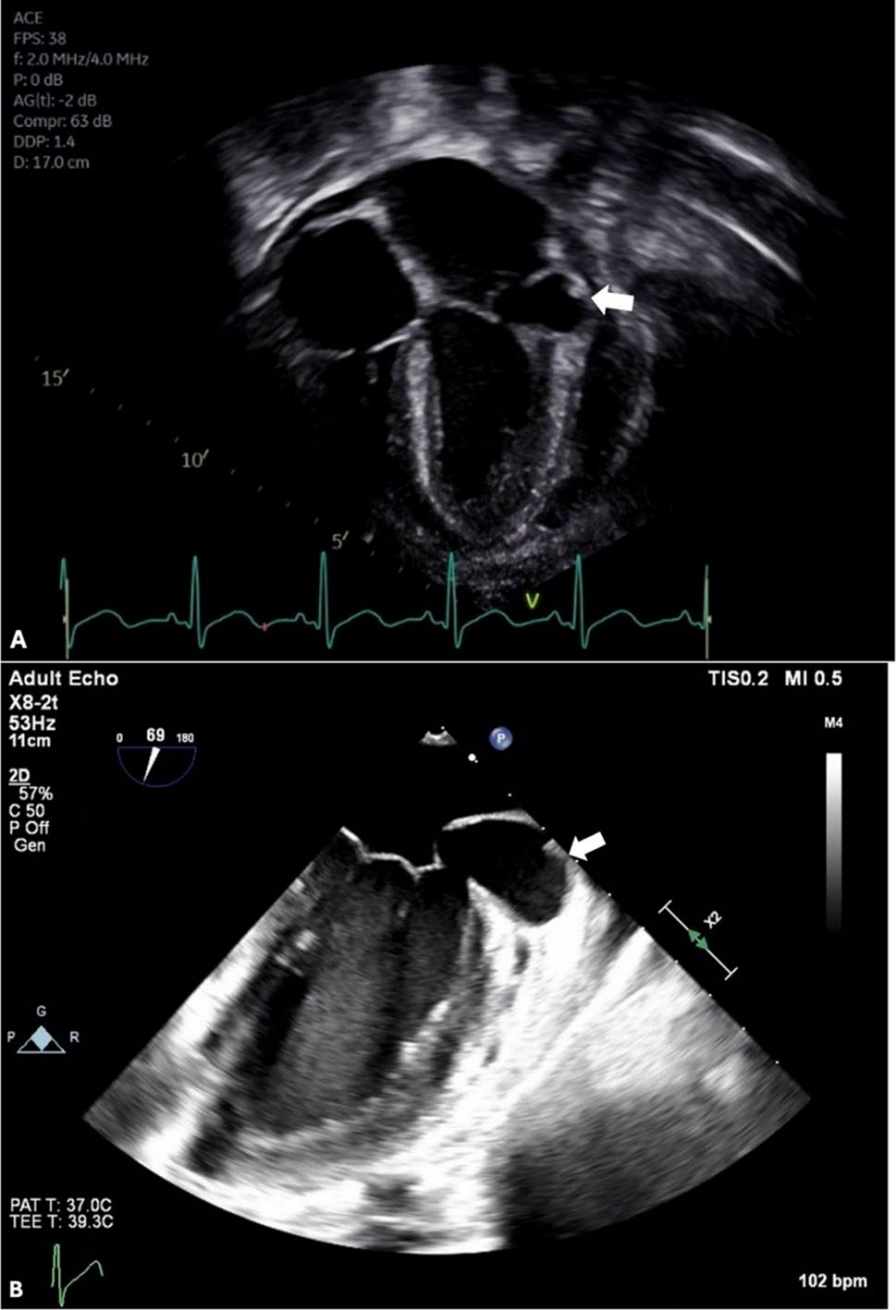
a Transthoracic echocardiogram demonstrating an aneurysm located underneath the posterior leaflet of the mitral valve near the mitral valve atrioventricular junction (arrow) b Transesophageal echocardiogram at the mid-esophageal two-chamber view demonstrating the 3.9 × 1.8 cm aneurysm as a result of dehiscence in the mitral valve atrioventricular junction fibrosus at the hinge point (arrow)
